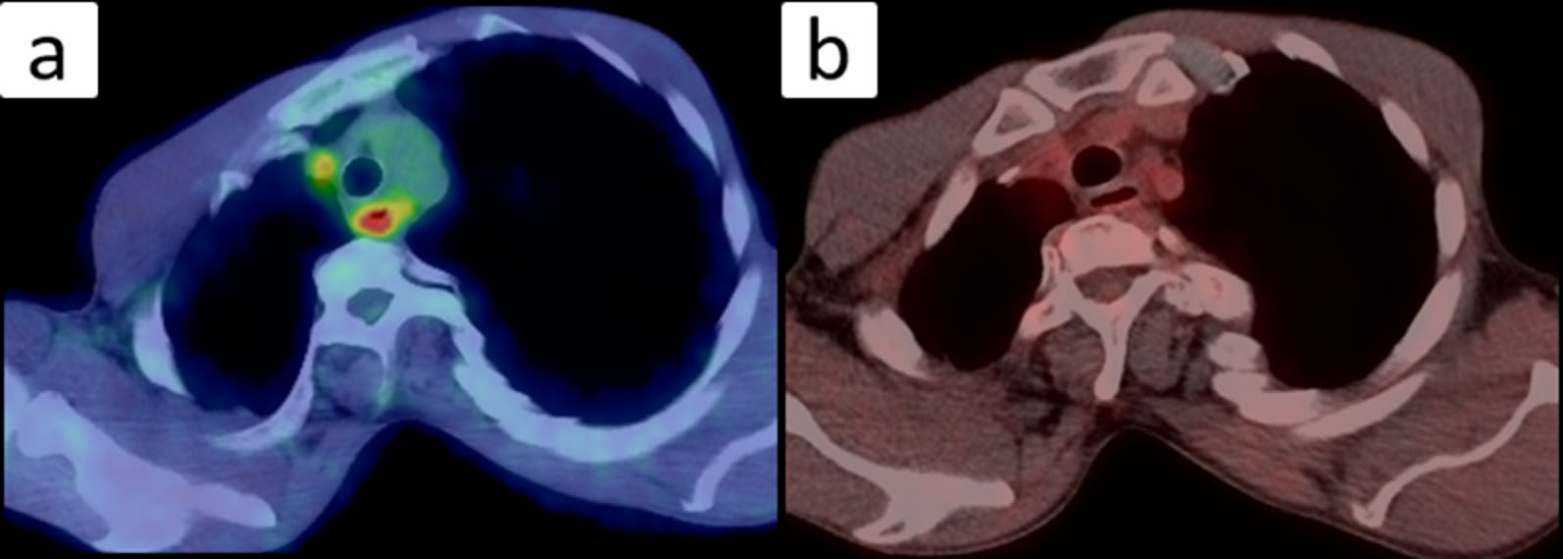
PET–CT images: a Before chemoradiotherapy, abnormal 18F-FDG accumulation was seen from the upper to middle thoracic esophagus, with an SUV max of 11.9. Mildly abnormal 18F-FDG accumulation was also seen in the lymph nodes around the right recurrent nerve. b After chemoradiotherapy, abnormal 18F-FDG accumulation remained only in the middle thoracic esophagus, with an SUV max of 3.87. Abnormal 18F-FDG accumulation in the lymph nodes had disappeared
